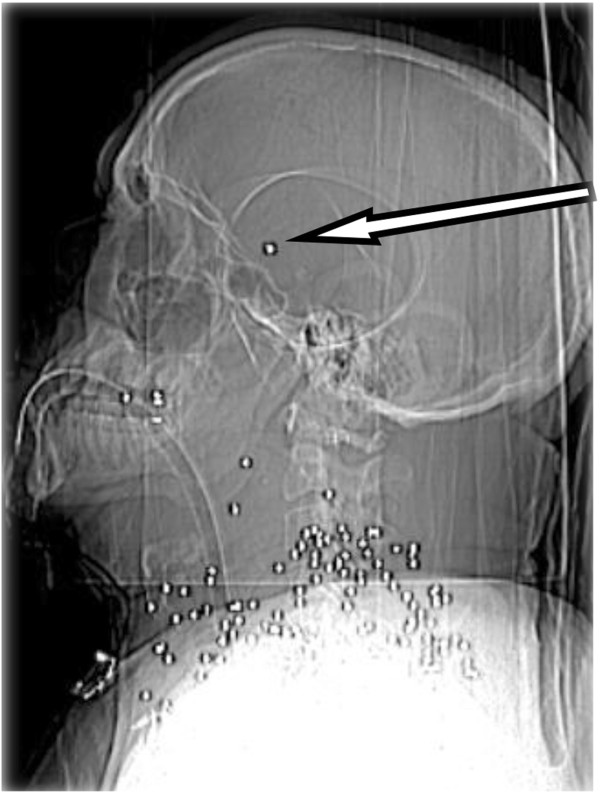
Lateral scout CT head and neck image at presentation.
